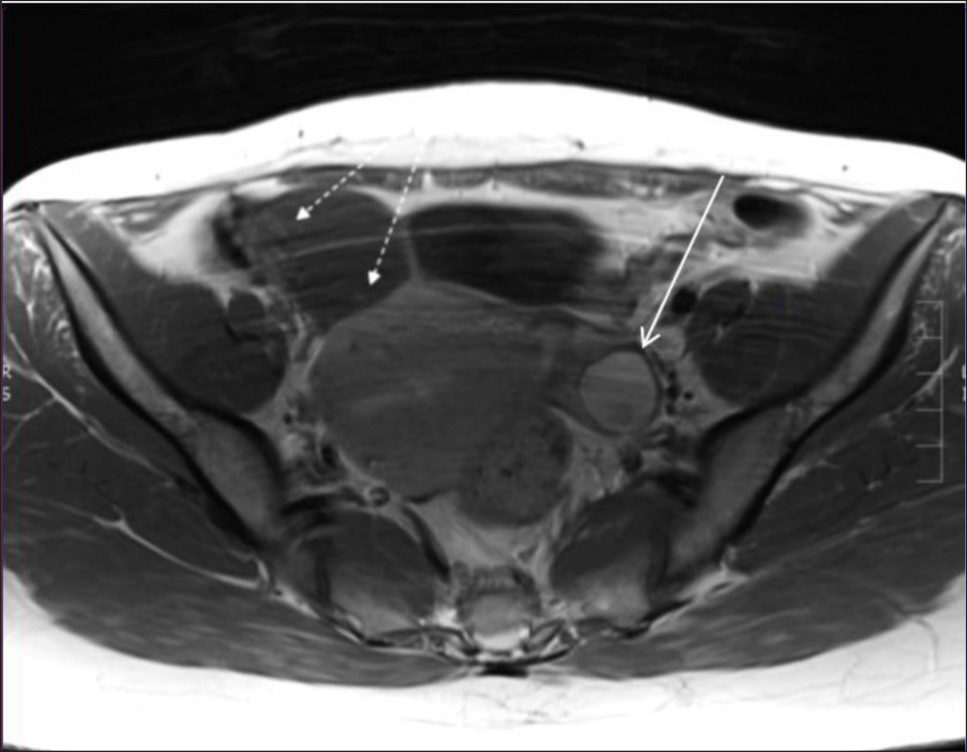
Axial contrast-enhanced T1-weighted MR.
